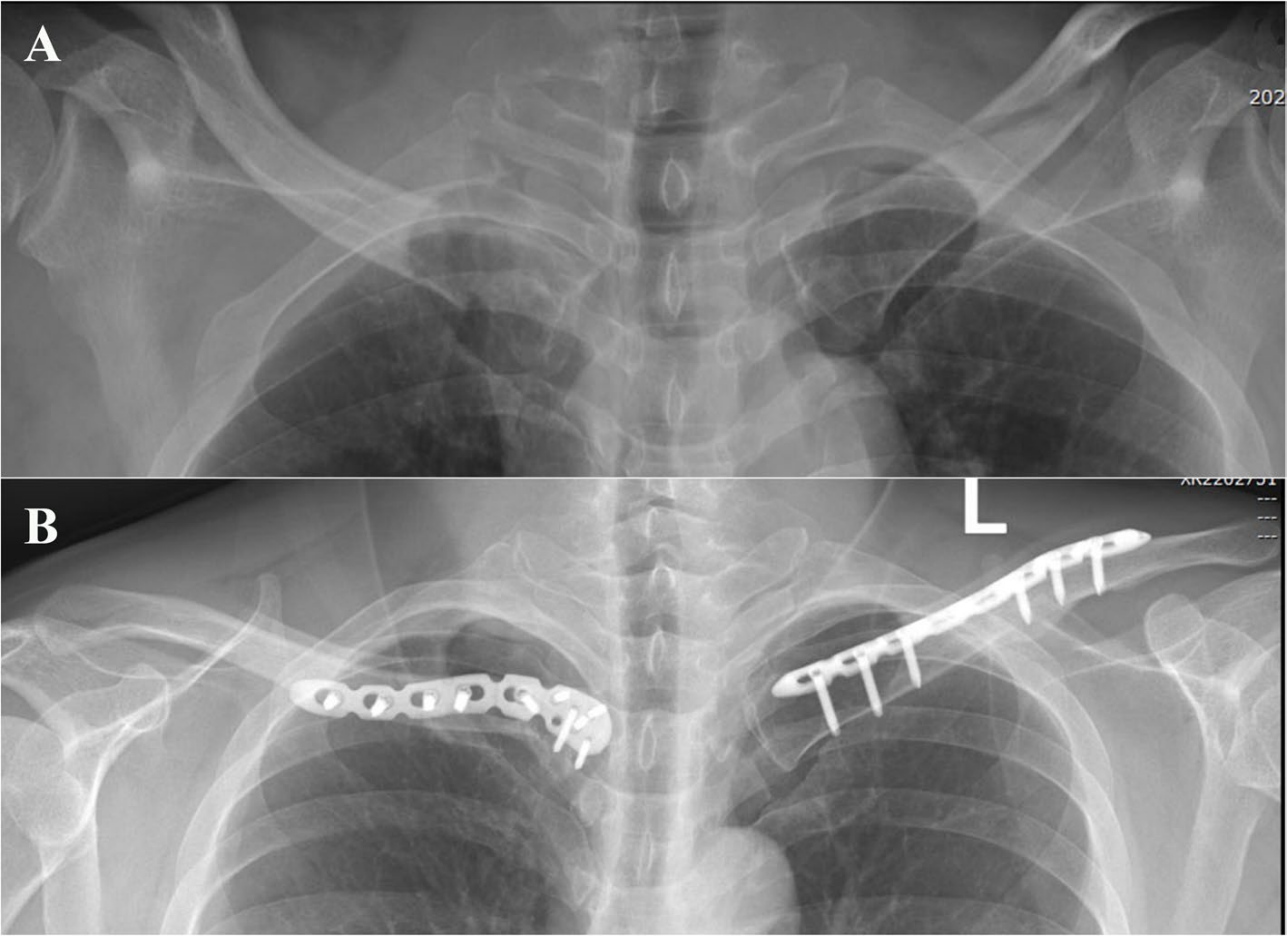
A Preoperative radiograph of a 53-year-old man showed a right intra-articular medial clavicle fracture and left mid-shaft clavicle fracture. B Radiograph 14 months postoperatively showed solid bone union on both sides after inverted distal clavicle plate fixation for the right clavicle fracture and reconstructive plate fixation for the left mid-clavicle fracture
